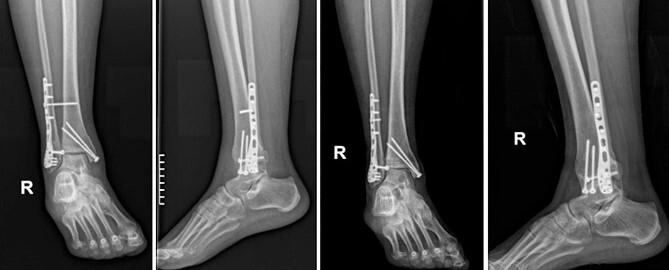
Anteroposterior and lateral X-ray of the ankle joint showing the broken syndesmotic screw and the replacement with a 3.5-mm cortical screw on the lateral malleolus 8 weeks after the initial surgery.
